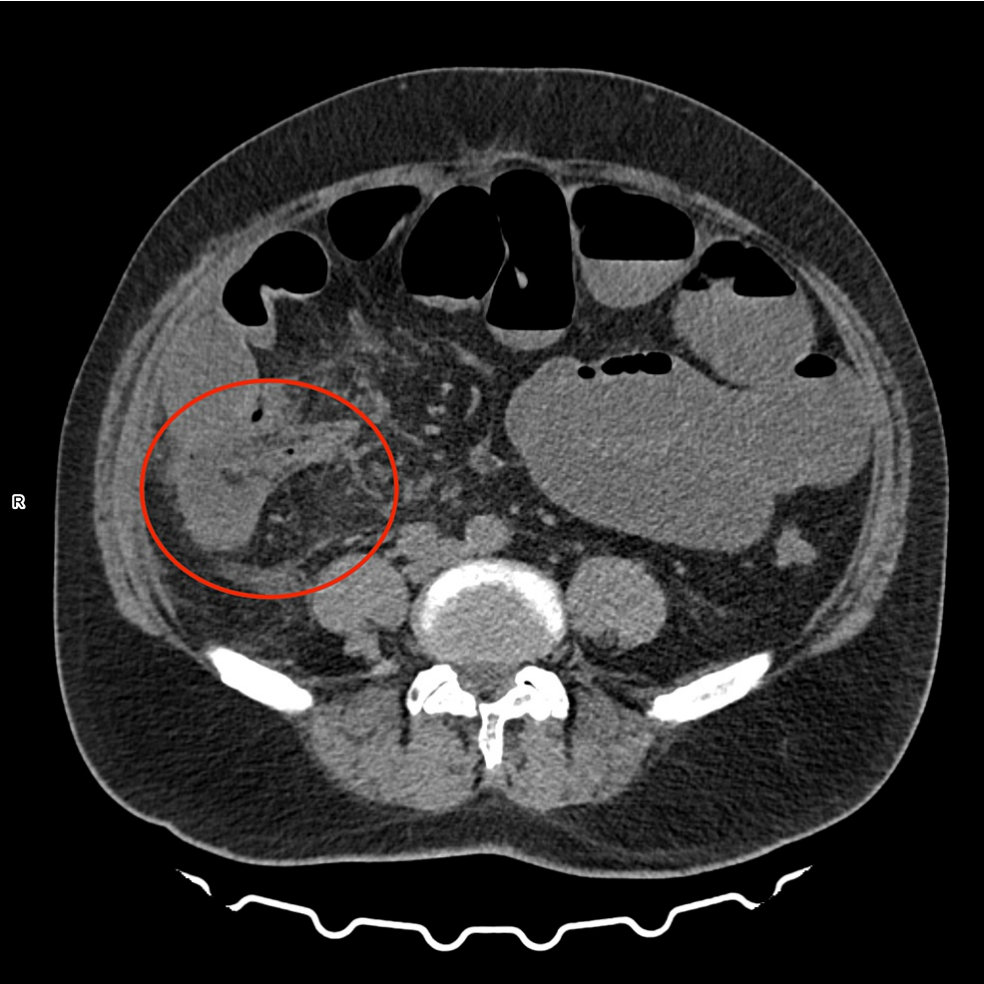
Appendix with inflammatory infiltrate Enlarged appendix to 16 mm (red circle, with fecaliths at its orifice - CT image corresponds to acute appendicitis. Inflammatory infiltration of the surrounding adipose tissue involving the cecum and terminal ileum. Small gas bubbles anterior to the inflamed appendix - likely periappendiceal abscess. Loop of the small intestine, mainly the jejunum, dilated to 52 mm, with a large amount of retained content and fluid levels - features of gastrointestinal obstruction.
